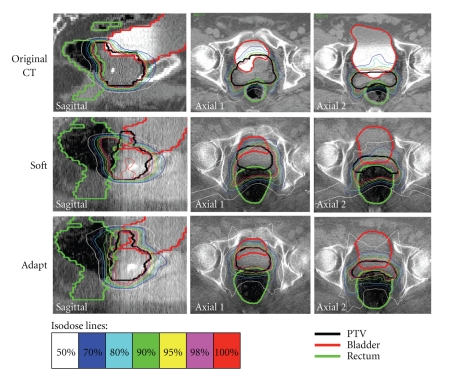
Isodose distribution comparison of the original CT plan (Original CT), the repositioned plan (Soft) and the adapted plan (Adapt) for one treatment fraction. Overlapping of PTV and OARs is caused by the manual expansion from CTV (prostate + rectum) with a 5 mm uniform margin.
